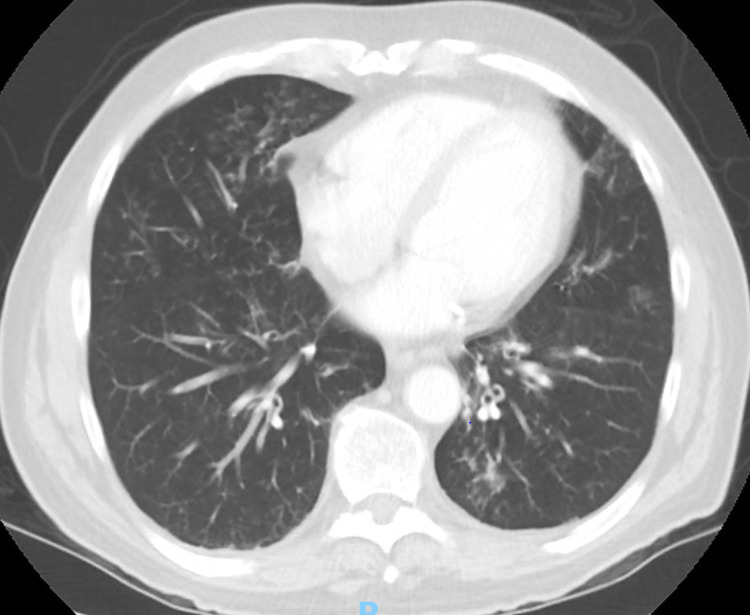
CT Chest with contrast showing mild to moderate peribronchial thickening, multiple foci of mucoid impaction, and ill-defined areas of ground-glass opacity, with tiny clustered nodules/tree-in-bud opacities and right middle lobe, lingular, and bilateral lower lobe predominance.
